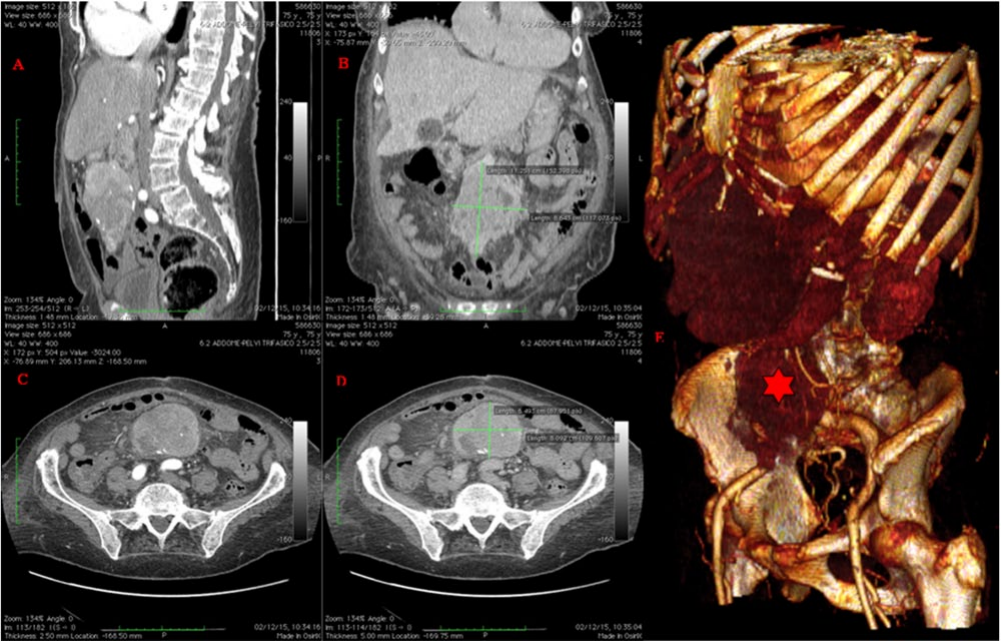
Computed tomography (CT) imaging. (A) Sagittal CT scan (late arterial phase, 35 seconds after bolus-tracking) showing a hypervascular tumor. (B) Coronal CT scan view (late portal phase at 70 seconds) showing slow washout of the primary lesion. (C) Axial CT scan view (arterial phase) confirming vascular enhancement and showing interaction with blood vessels. (D) Axial CT scan view (portal phase). (E) 3D volume rendering reconstruction: the neuroendocrine tumor (red star) is shown.
